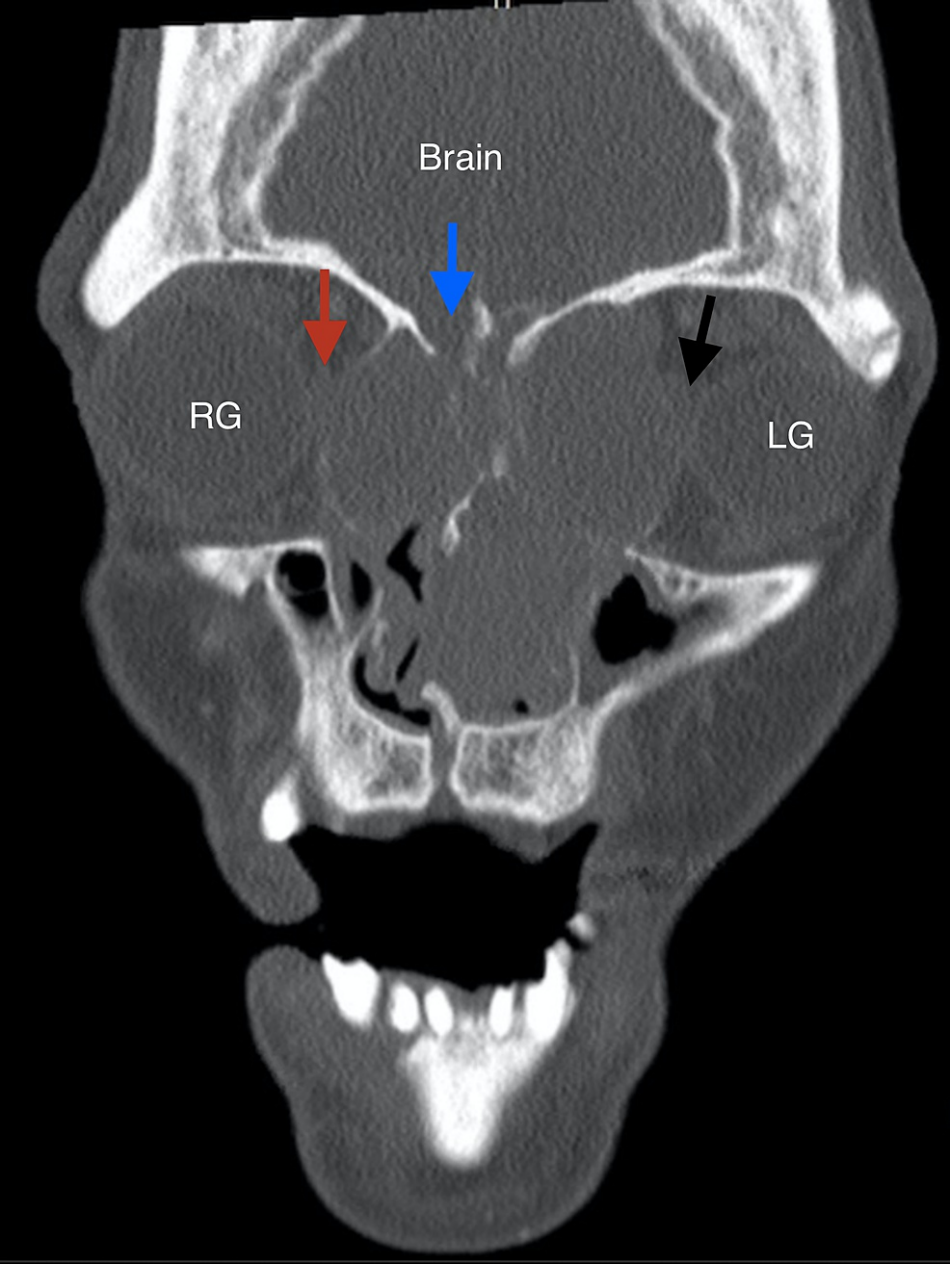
CT bone window, coronal view, at the level of the orbit, showing proptosis of bilateral globes. The red arrow indicates the invasion of the right orbital cavity, which had lateralized the right medial orbital wall, causing proptosis of the right globe (RG). The black arrow indicates similar invasion of the left orbit, with erosion of the left medial orbital wall and proptosis of the left globe (LG). The blue arrow indicates erosion of the anterior skull base, lateral to the cribriform plate.
